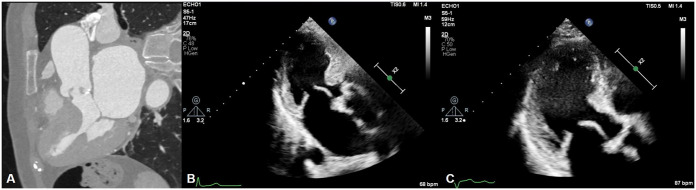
Comparison between (A) preoperative cardiac computed tomography, (B) pre- and (C) postoperative echocardiography (parasternal long axis view).
